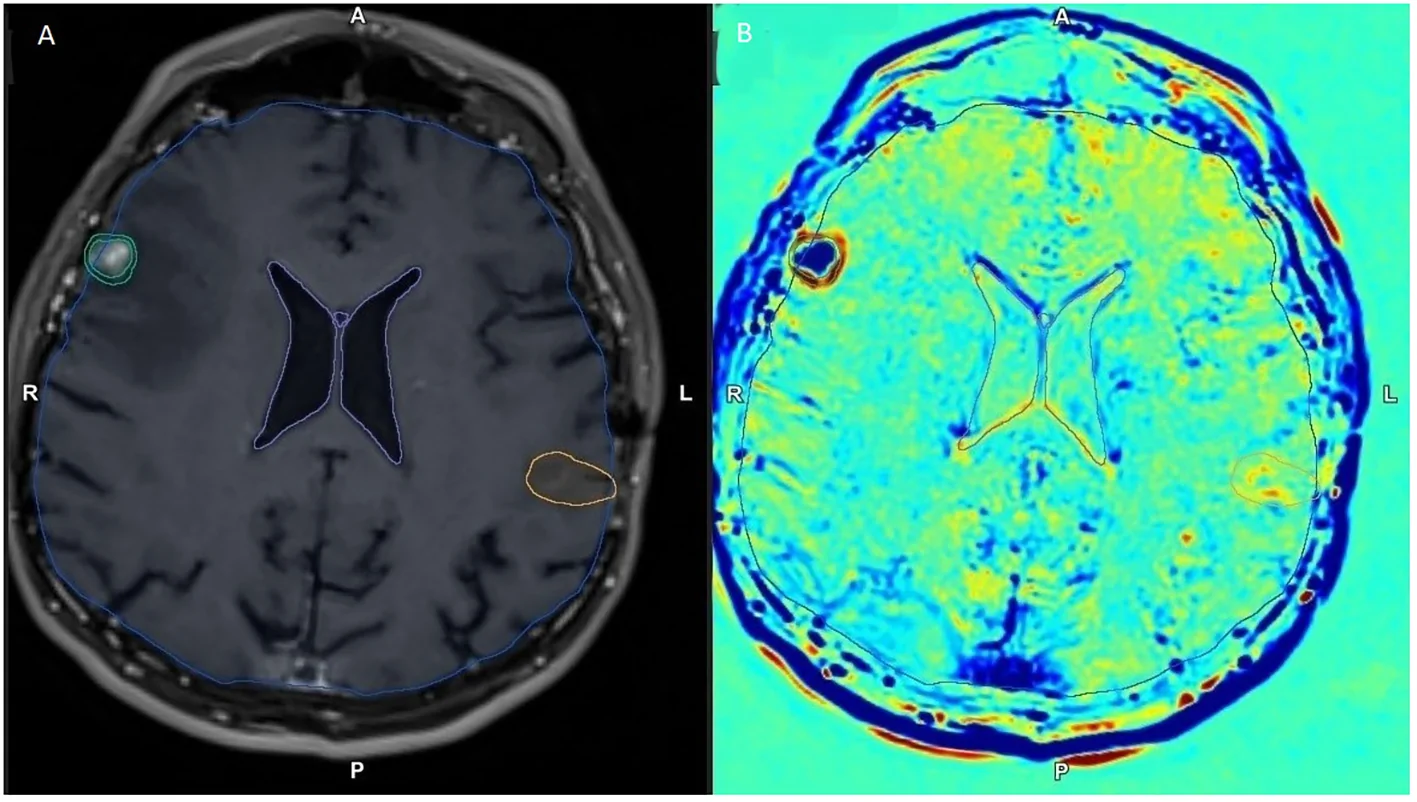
Post-operative TRAMs/CCA findings demonstrating the absence of early contrast clearance and delayed contrast retention within the resection cavity (area marked in orange). (A) Contrast-enhanced T1-weighted MRI acquired for treatment planning showing post-operative enhancement of the resection cavity. (B) TRAMs/CCA demonstrating the absence of early contrast clearance and delayed contrast retention 8 months post-surgery. Additional active tumor identified in the right frontal lobe (area marked in green).
